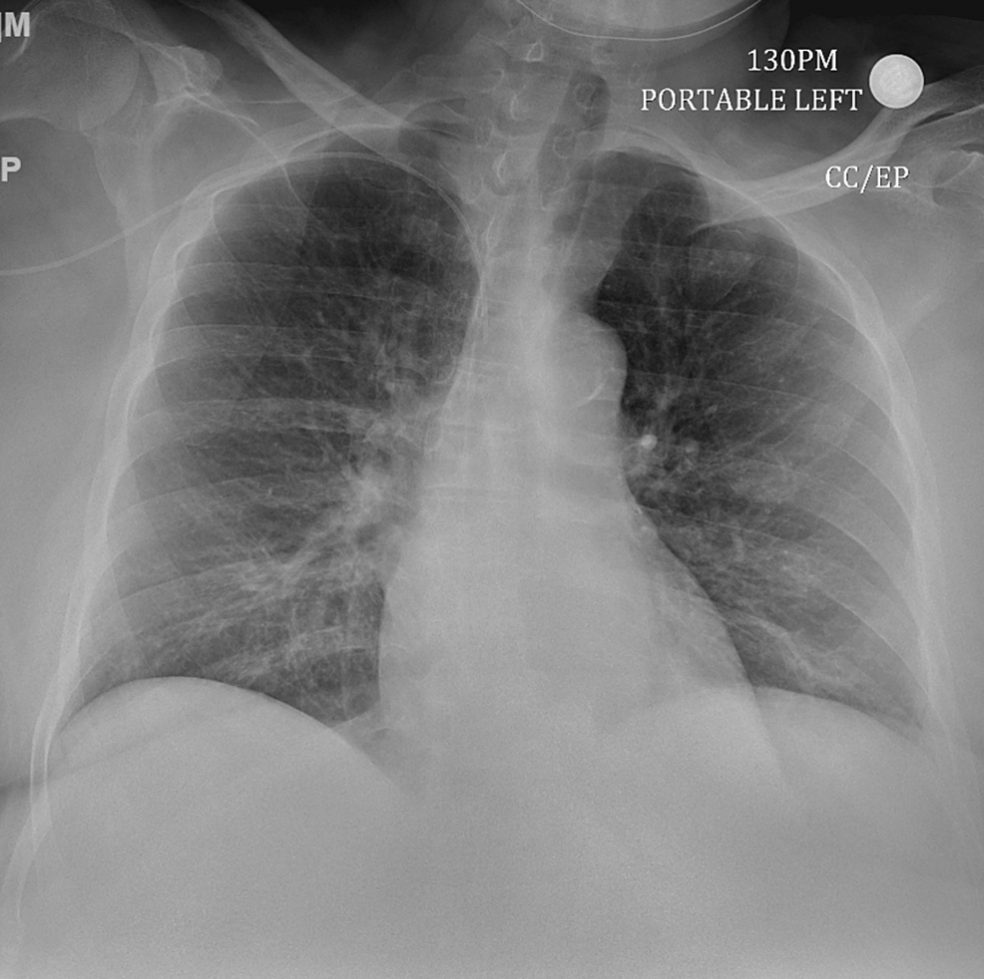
Chest X-ray at admission negative for cardiopulmonary abnormality
